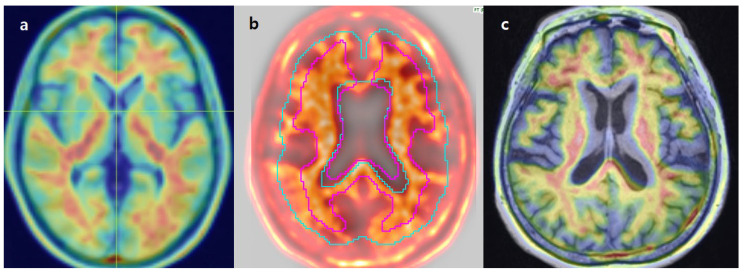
Registration of PET images on BTXBrain (a), MIM (b), and SCALE PET (c). In SCALE PET, PET images were misaligned with the anatomical MR image, while BTXBrain and MIM demonstrated proper alignment.
